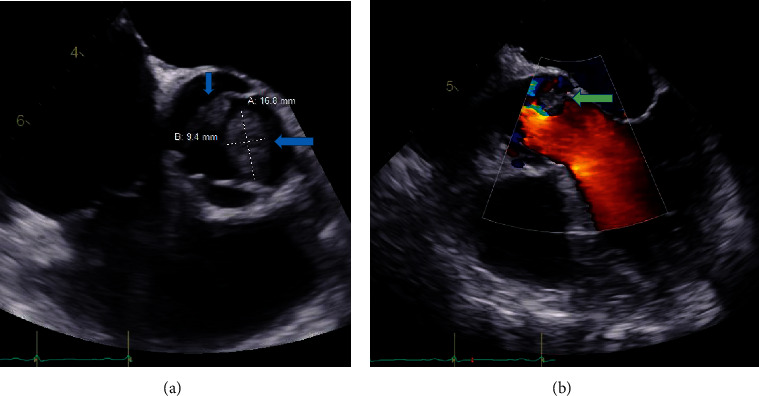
(a) TEE image showed large vegetation (large blue arrow) and another small vegetation (small blue arrow). (b) TEE color Doppler showed AV regurgitation and the vegetation over the valve leaflet (green arrow).
